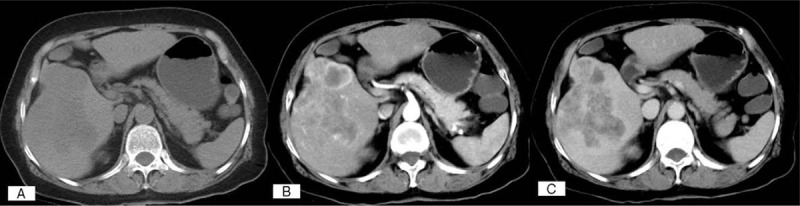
Dynamic CT scans of a PHNEN (G2), which exhibits early arterial enhancement followed by wash-out in the venous phase, similar to a typical HCC.
